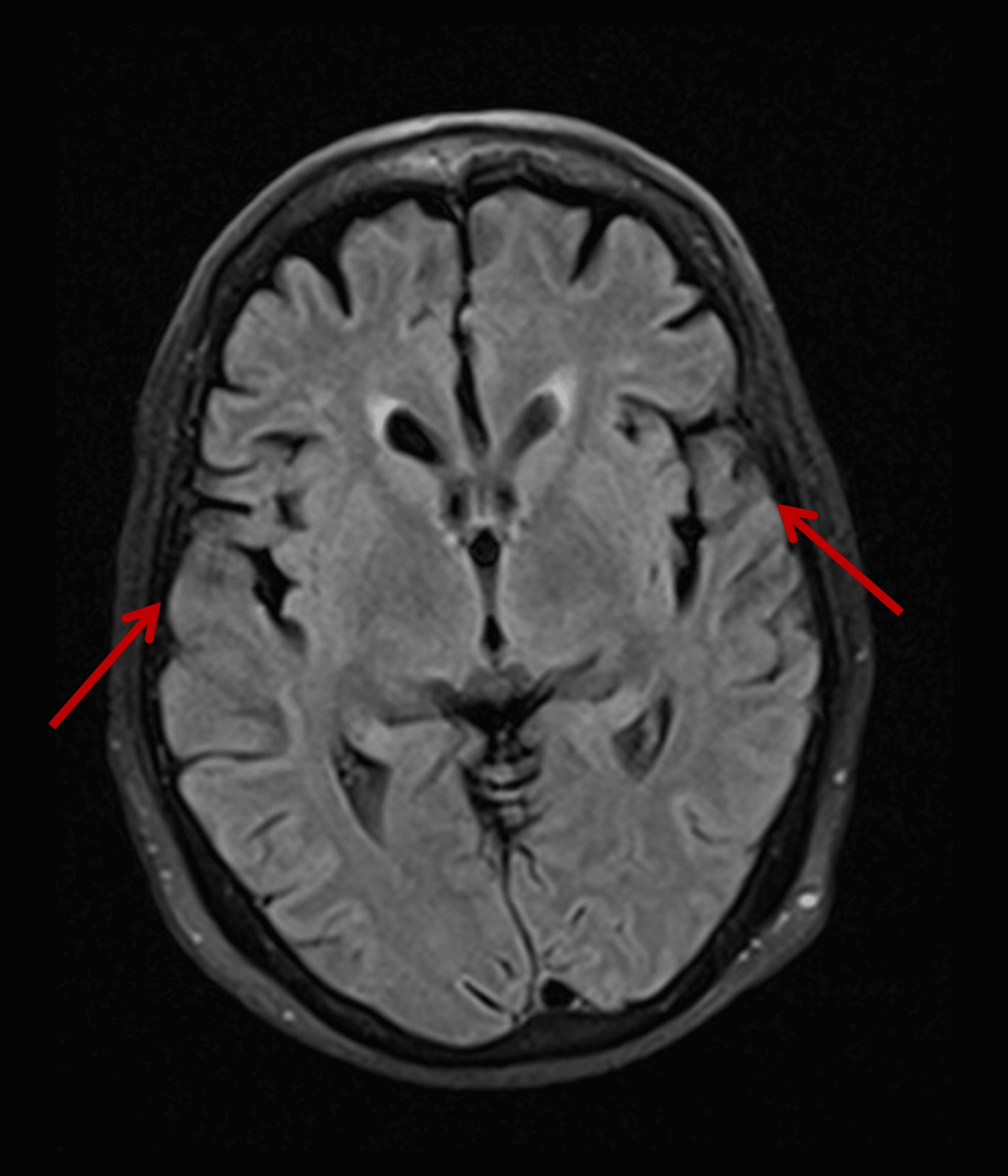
Brain MRI showing temporo-insular alterations compatible with Parkinson's disease (arrows)
